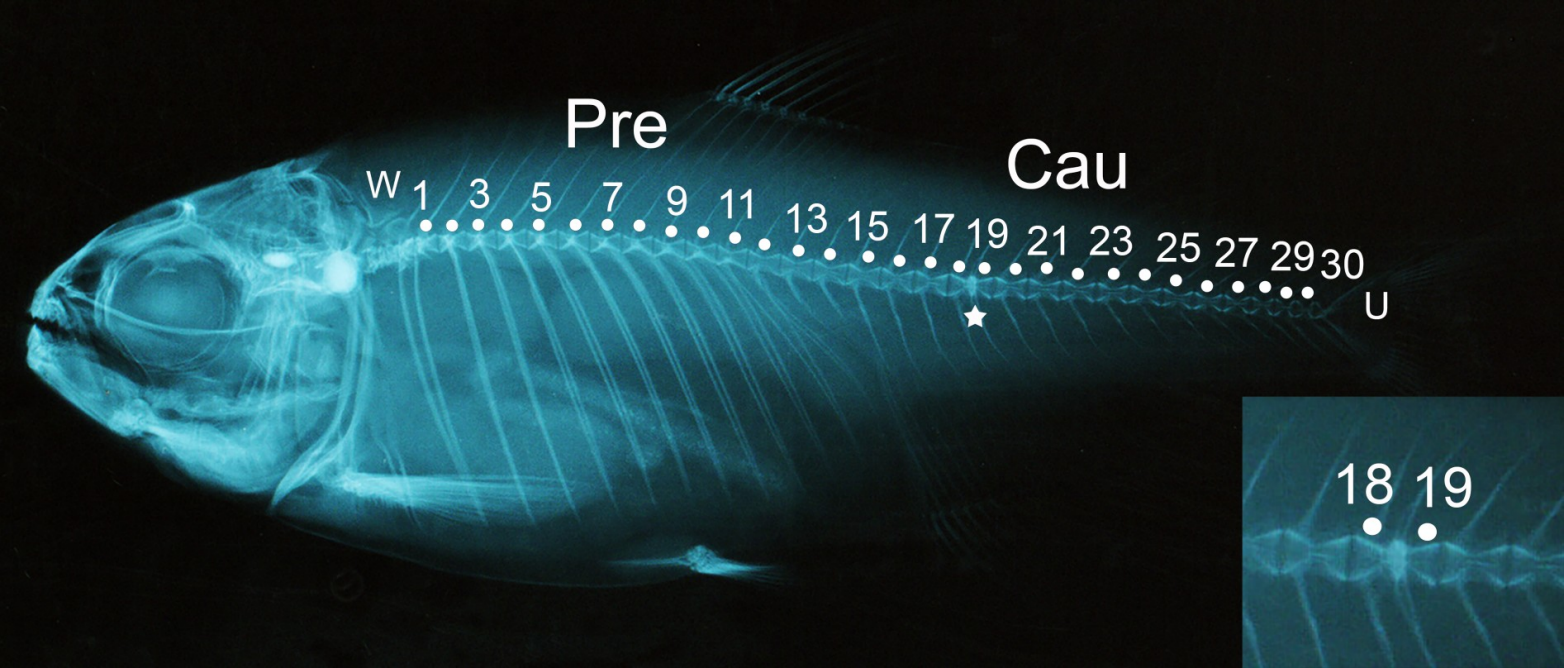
X-ray of *Astyanax mexicanus* indicating method for counting vertebrae and major anatomical structures. Vertebrae were labelled individually with points but only odd numbers are included to avoid clutter (except for the last vertebra, number 30). Four major regions were defined in the counts. 1) W: Weberian apparatus. 2) Pre: Precaudal vertebrae, include the vertebrae with ribs (1–12). 3) Cau: Caudal vertebrae include vertebrae with hemal spines (13–30). 4) U: Urostyle. The star indicates a fused vertebra that was counted as vertebrae 18 and 19. Notice the extra hemal spines and the junction formed in the middle of the vertebral body. Bottom right insert: an amplified view of the fused vertebra. The Weberian apparatus and the urostyle were not included in the counts.
